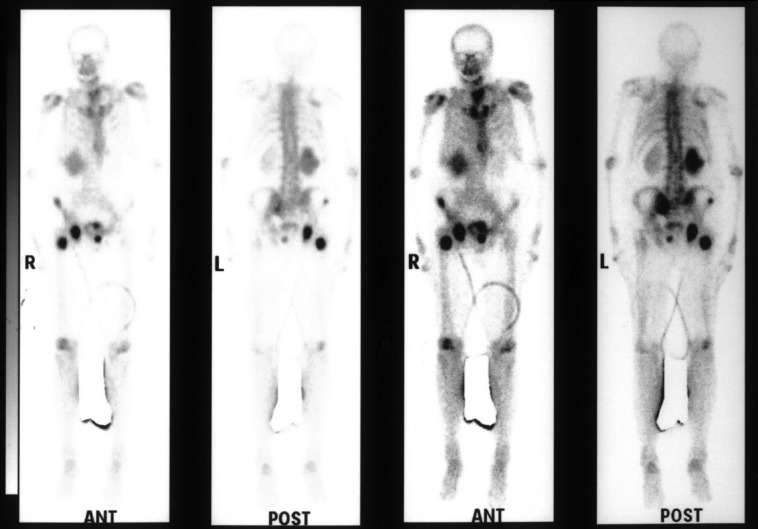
Single-photon emission computed tomography scan with ^99m^Tc-MDP demonstrated that the patient exhibited systemic bone metastasis involving the bilateral ilium, right acetabular, right pubis and right femoral.
